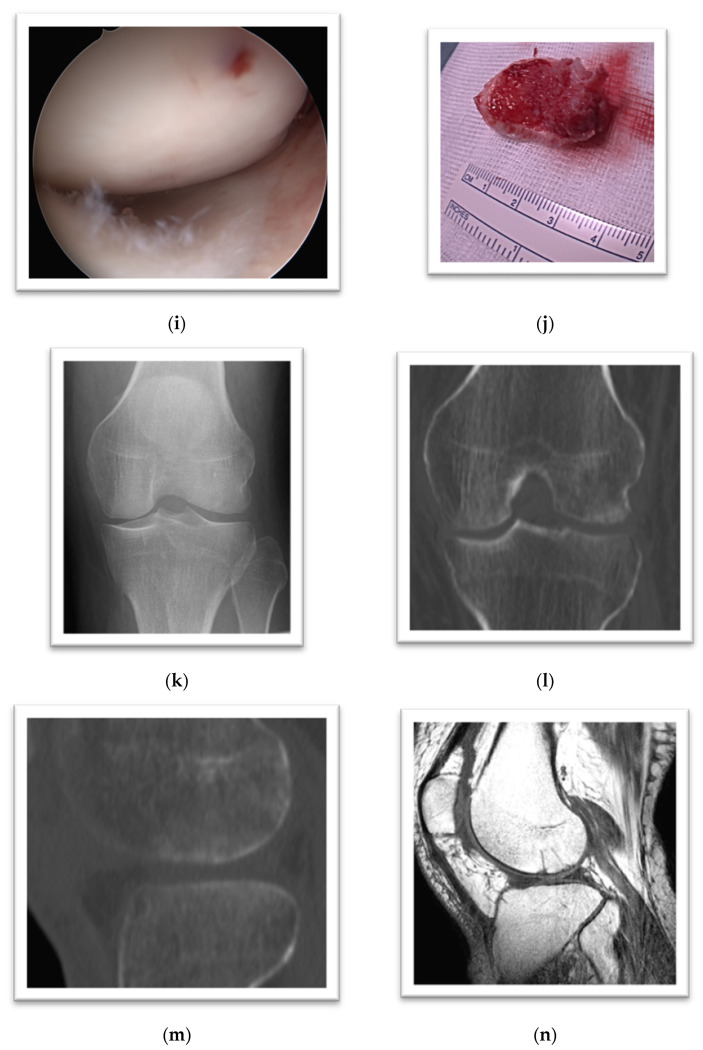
(a) Anterior-posterior radiograph of the left knee, limited range of motion due to the injury is obvious. (b) Lateral radiograph of the left knee, the osteochondral fragment is located inferior to the lower pole of the patella. (c–e) The coronal, axial and sagittal reconstruction during computed tomography (CT) reveals a massive osteochondral fracture of the lateral femoral condyle (LFC). (f) Arthroscopic evaluation of the displaced fragment. (g) The majority of the weight-bearing portion of the lateral femoral condyle is involved. (h) Reconstruction of the LFC after fixation with multiple PLLA darts. (i) Arthroscopic evaluation after internal fixation. (j) Osteochondral fragment with surface area measuring 3 × 2.8 cm, debridement was performed ex situ. (k) Postoperative AP radiograph shows anatomic articular alignment. (l,m) Sagittal and coronal CT reconstruction after three months shows adequate healing, but some subchondral sclerosis is present. (n) Sagittal MRI (T1) follow-up at six months, intact and vital cartilage surface, remaining small subchondral lesions at the sites of dart insertion.
